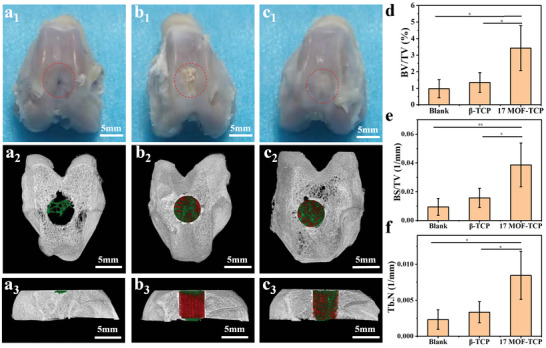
The in vivo regeneration effects of the osteochondral defects treated with different scaffolds. Digital photographs (a1–c1), the transverse view images (a2–c2), and sagittal view images (a3–c3) of the osteochondral defects reconstructed by microcomputed tomography (Micro‐CT). The green, red, and gray‐white color in Micro‐CT images represent new bone, scaffold, and native bone, respectively. The newly formed bone assessment including d) the ratio of new bone volume to defect volume (BV/TV), e) bone surface mineral density (BS/TV), f) the trabecular number of the new bone (Tb. N) after 12 weeks of implantation (*n* = 5). p* < 0.05, *p* < 0.01, p* < 0.001.
